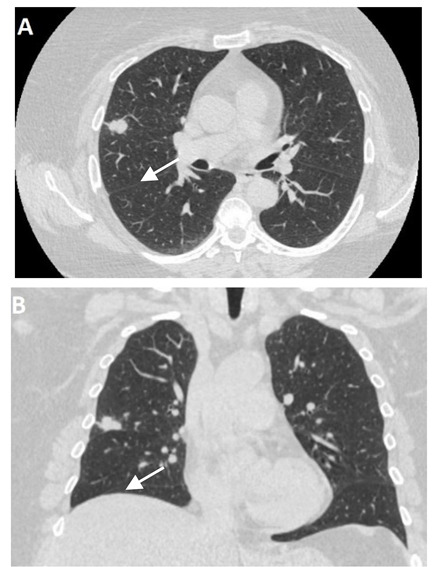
CT chest axial (image A) and coronal (image B) showing well-defined 2 cm nodular lesion in the Right Middle Lobe (RML) denoted by an arrow.
